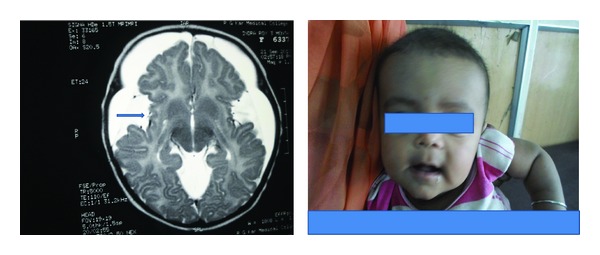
The child with a large head and his MRI brain revealing wide CSF spaces with temporal lobe hypoplasia, bilateral front parietal subdural effusions and dilatation of the sylvian fissures with open opercula (arrow), and high signal intensity seen in bilateral caudate nuclei, putamen, and deep subcortical white matter. Widening of the sylvian fissure gives the characteristic “bat-wing” appearance.
